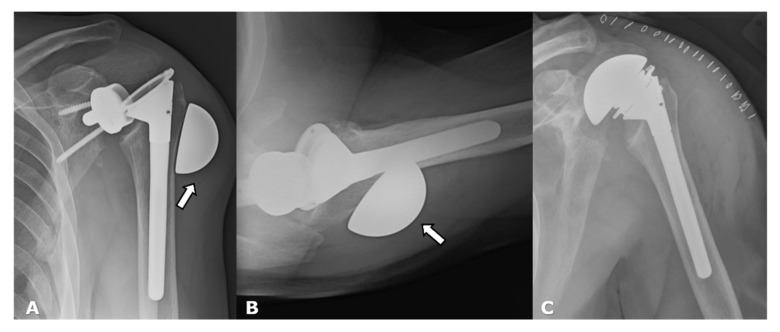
(A) Frontal and (B) axillary radiographs in a patient with RTSA shows dissociation of the glenosphere (arrows) from the metaglene, with displacement of the glenosphere into the posterior soft tissues. (C). The patient was treated via conversion to salvage HA.
